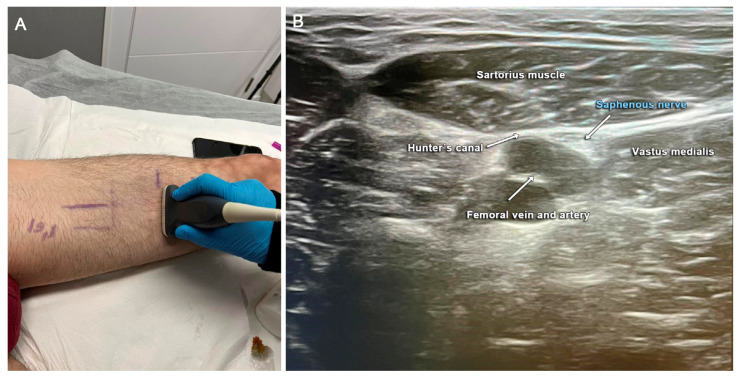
Preoperative images of the case. (A) To obtain ultrasound (US) images, the transducer was placed horizontally on the proximal medial thigh to target the adductor canal. (B) US image showing how the canal is bordered by the sartorius muscle superficially, the vastus medialis muscle laterally, and the adductor longus muscle deeply. The saphenous nerve appears as a hyperechoic oval structure situated inside the canal with the femoral artery and vein.
